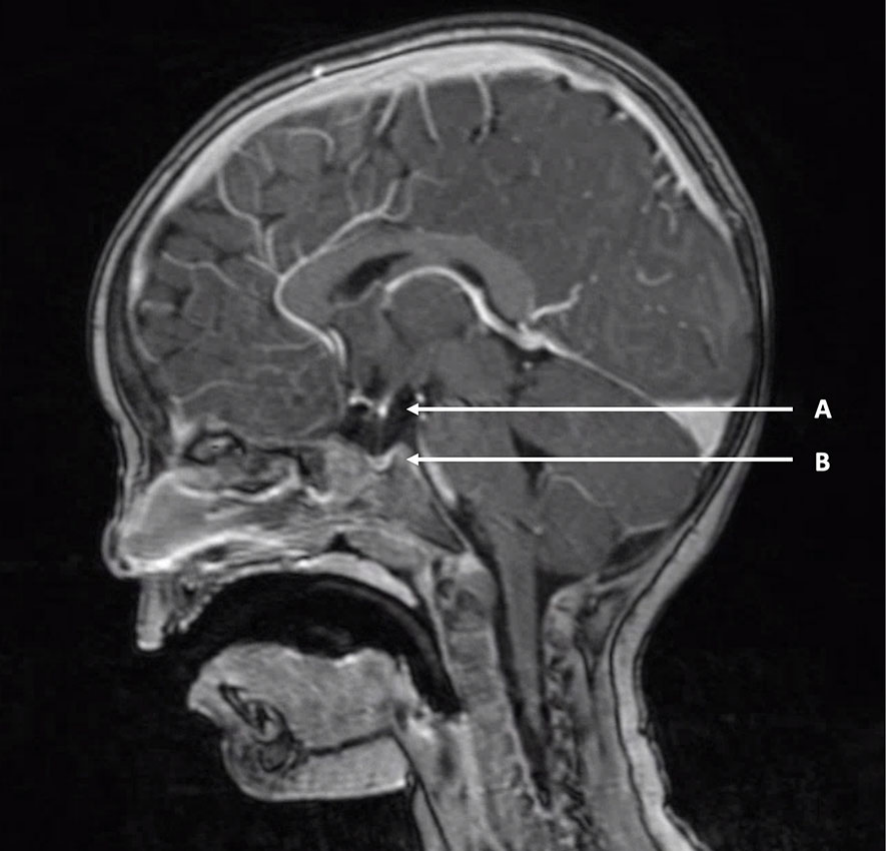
Example of pituitary stalk interruption syndrome. T1-weighted MRI of a two-year-old boy with pituitary stalk interruption syndrome. (A) “Bright white spot” of ectopic posterior pituitary positioned at the hypothalamic base. (B) Hypoplastic anterior pituitary in the sella turcica. A thin pituitary stalk is visible between (A, B).
